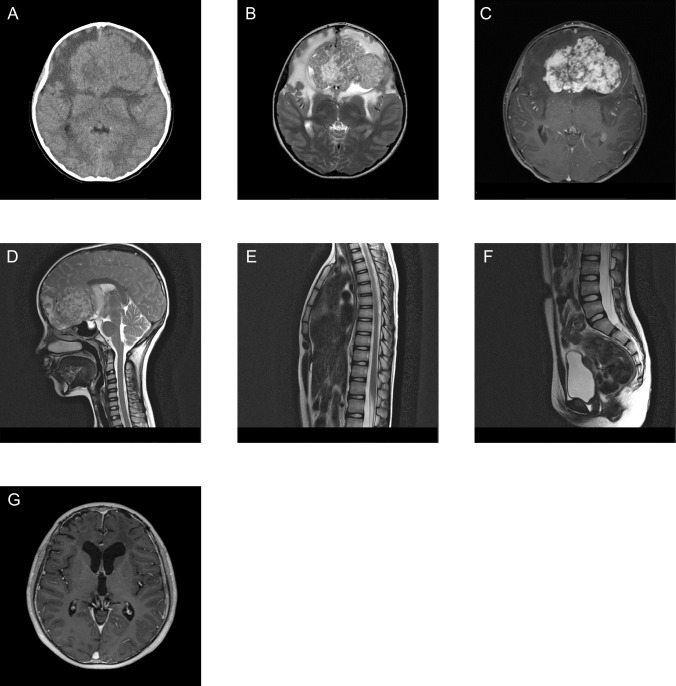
Head computed tomography (CT) scan showing a bilateral frontal mass without calcification A. Head magnetic resonance (MR) imaging demonstrates significant peritumoral edema on T2-weighted images and heterogeneous enhancement on gadolinium-enhanced T1-weighted images B, C. Whole-spine MR imaging shows no evidence of spinal dissemination D-F. A gross total resection was confirmed on gadolinium-enhanced T1-weighted images (G)
